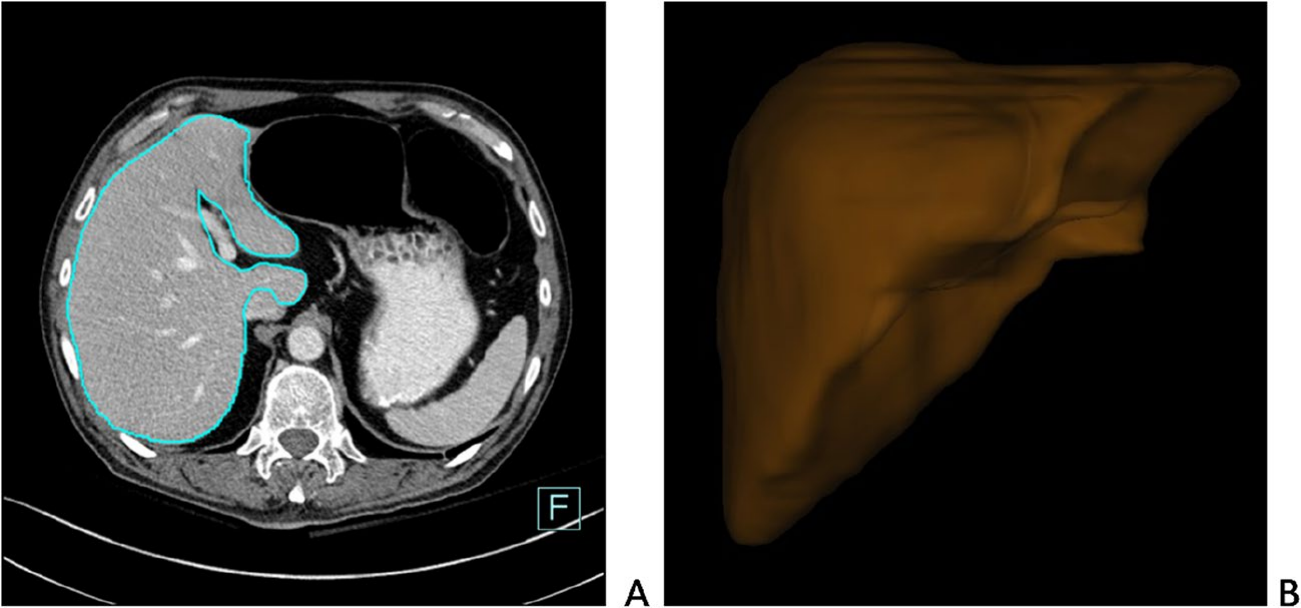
Refined contour of the liver after semi-automatic extraction of the liver parenchyma from abdominal CT-image in Syngo.Via and excluding the hepatic and portal vein (A) 3D model of the liver after post-processed liver shape (B).
